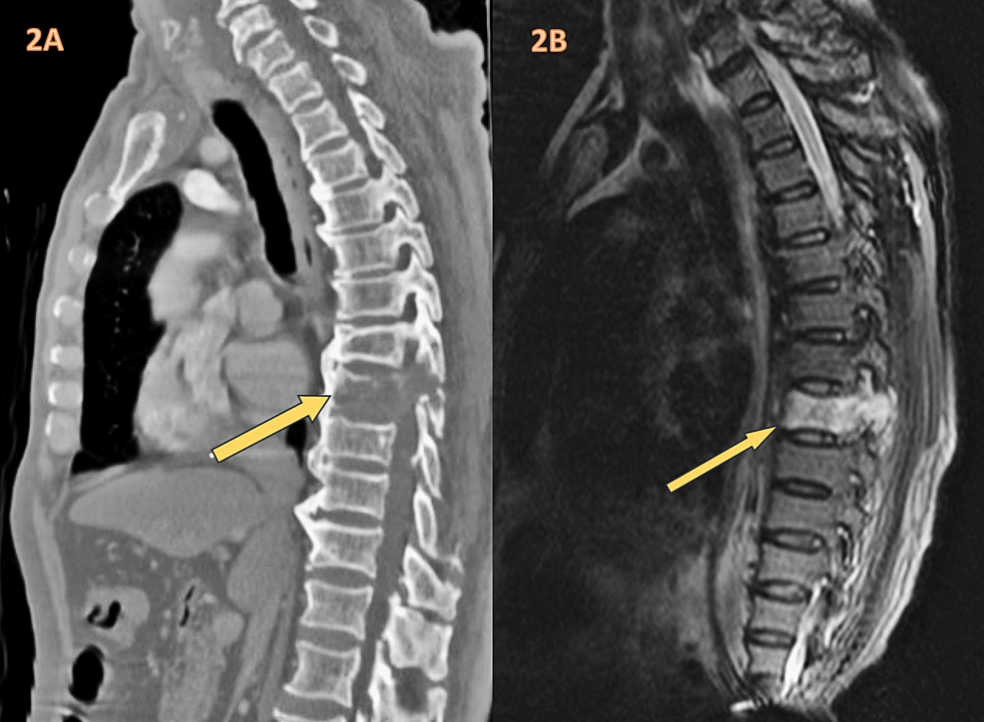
(A) Axial spine CT scan revealing metastatic lesion and (B) axial spine MRI displaying metastatic lesion. CT: computer tomography, MRI: magnetic resonance imaging.
